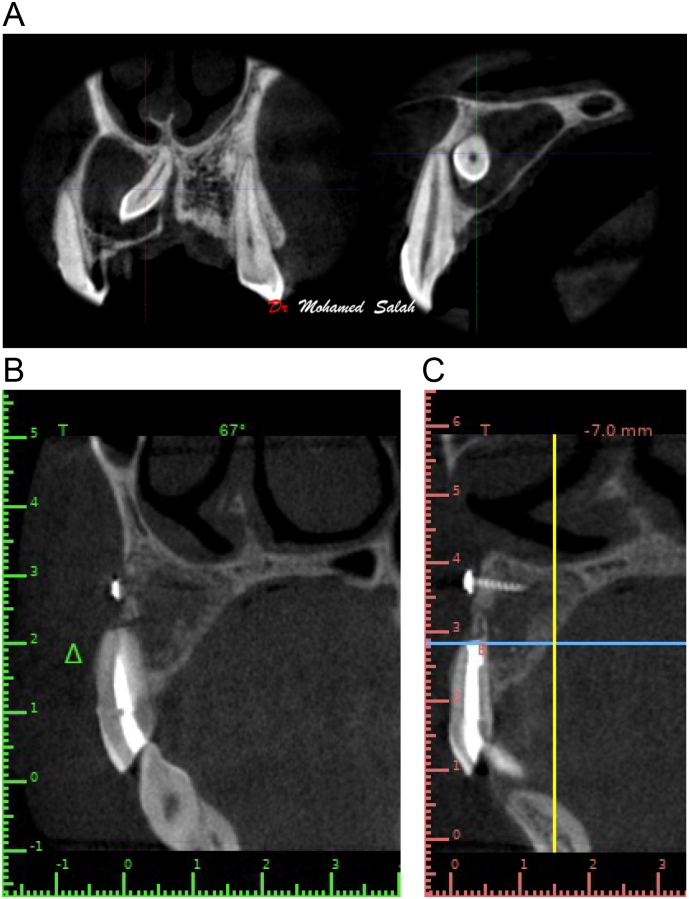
(A) Pre-operative CBCT. (B),(C) 6-month post-operative CBCT
